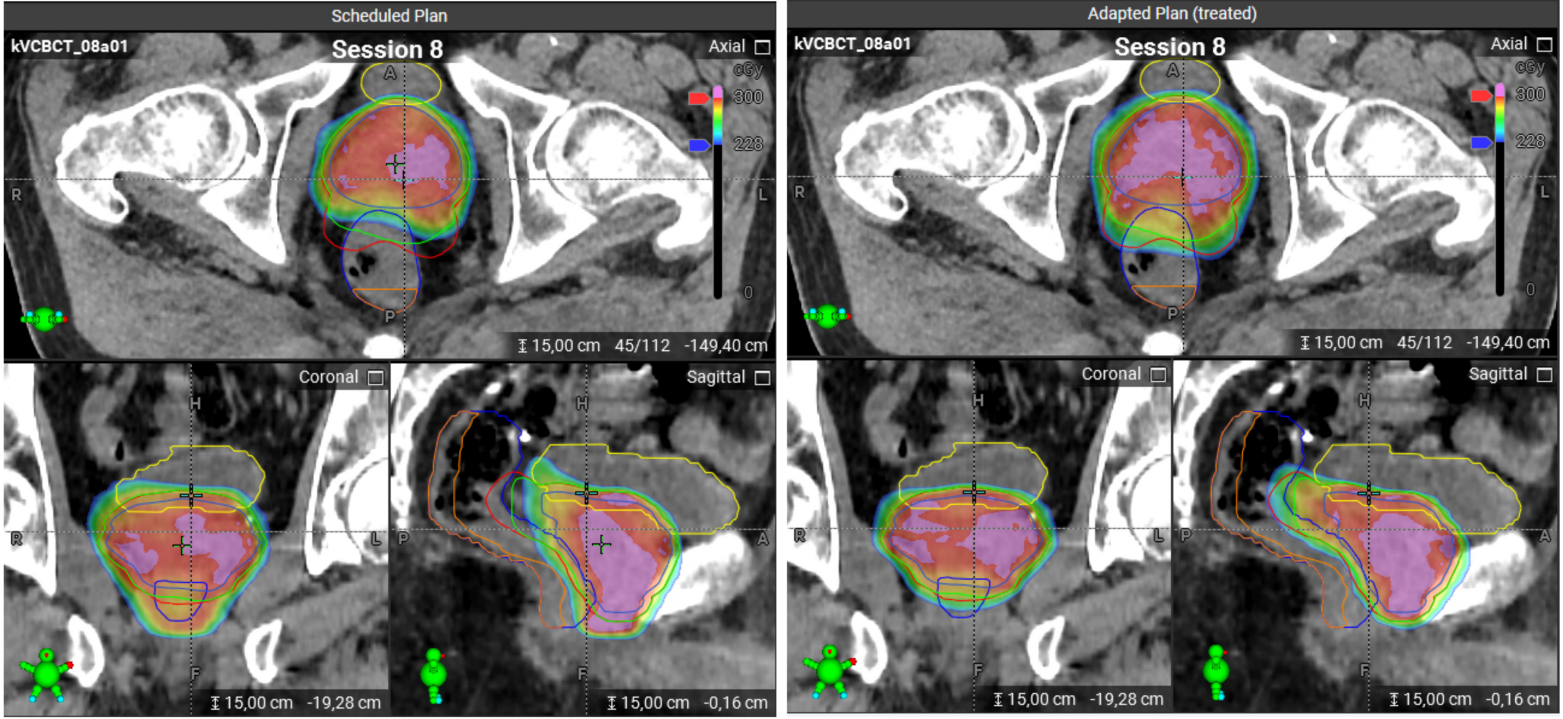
Scheduled and adapted plans on a CBCT image for the same treatment session. Left panel: The scheduled plan. Right panel: The adapted plan. The color scheme for the contours: bladder – yellow, rectum – dark blue, PRW – orange, PTV/SIB1/SIB2 – red/green/blue. The dose distributions are visualized using a color wash, where blue corresponds to 2.28 Gy and red to 3 Gy. Doses above 3 Gy are indicated in pink. The scheduled plan shows strong underdosage for PTV and SIB1 which could be compensated with the adapted plan, as can be seen in sagittal and axial views.
